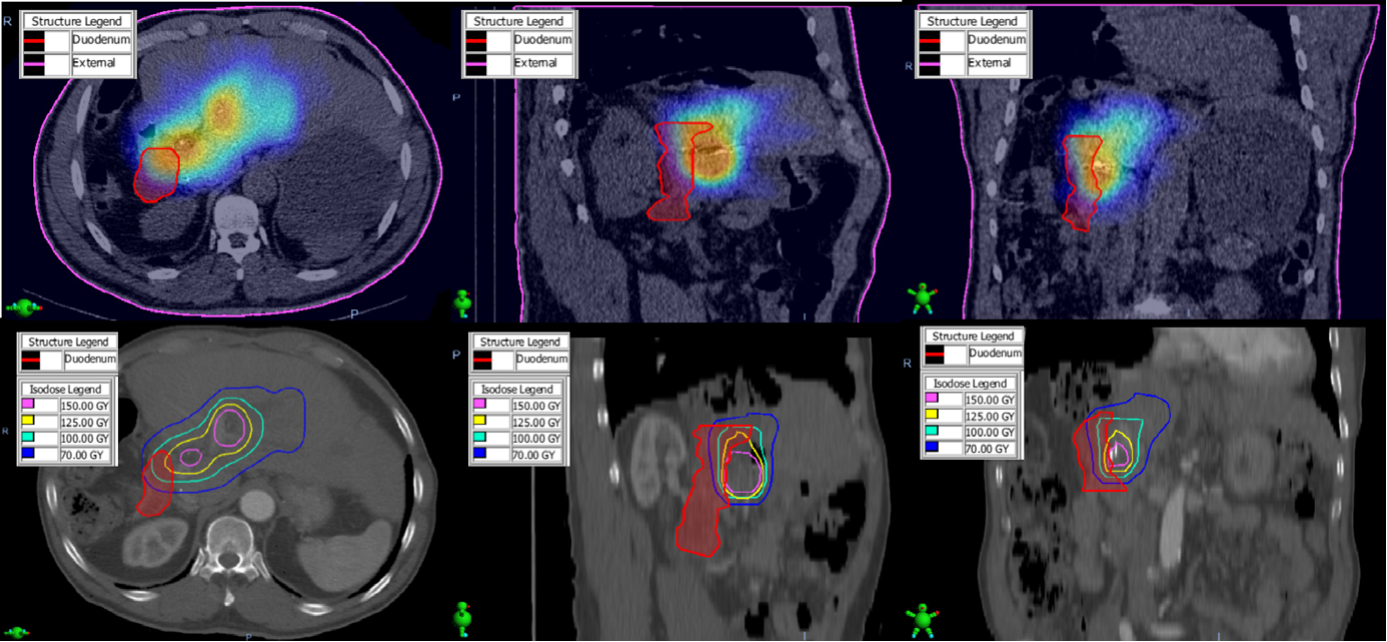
The deformably registered post‐treatment SPECT/CT (top), and the 11‐week follow‐up CT (bottom) with the superimposed ^90^Y isodose distribution for Case #3. Notice the prescription isodose volume (125 Gy) extends outside the liver into the duodenum.
